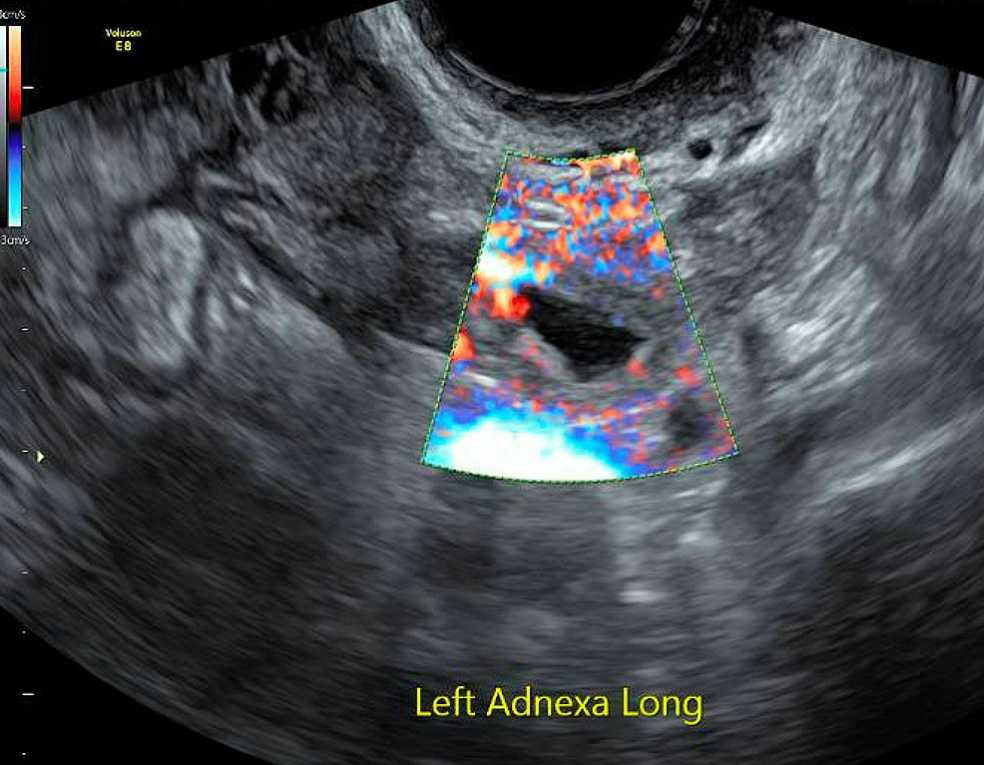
Left adnexal mass on transvaginal ultrasound
